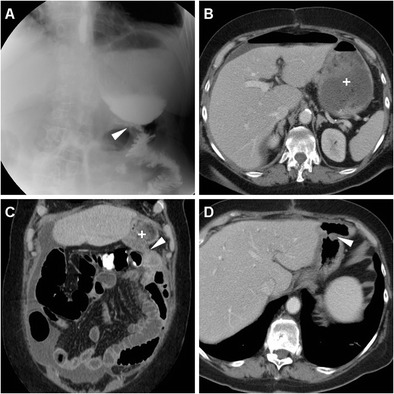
A 77-year-old female with distal gastric carcinoma underwent partial gastrectomy. Early postoperative CF (A) showed slow emptying of gastric remnant through the GJS (arrowhead), without appreciable extraluminal leaks. Six days later, the patient suffered from high fever: contrast-enhanced CT (B-C) showed dilated remnant stomach (+), absence of collections adjacent to the GJS (arrowheads in C and D), but persistent intraperitoneal air and fluid consistent with peritonitis, confirmed at surgical exploration: after redo anastomosis, the final status of GJS (arrowhead) is shown in D
